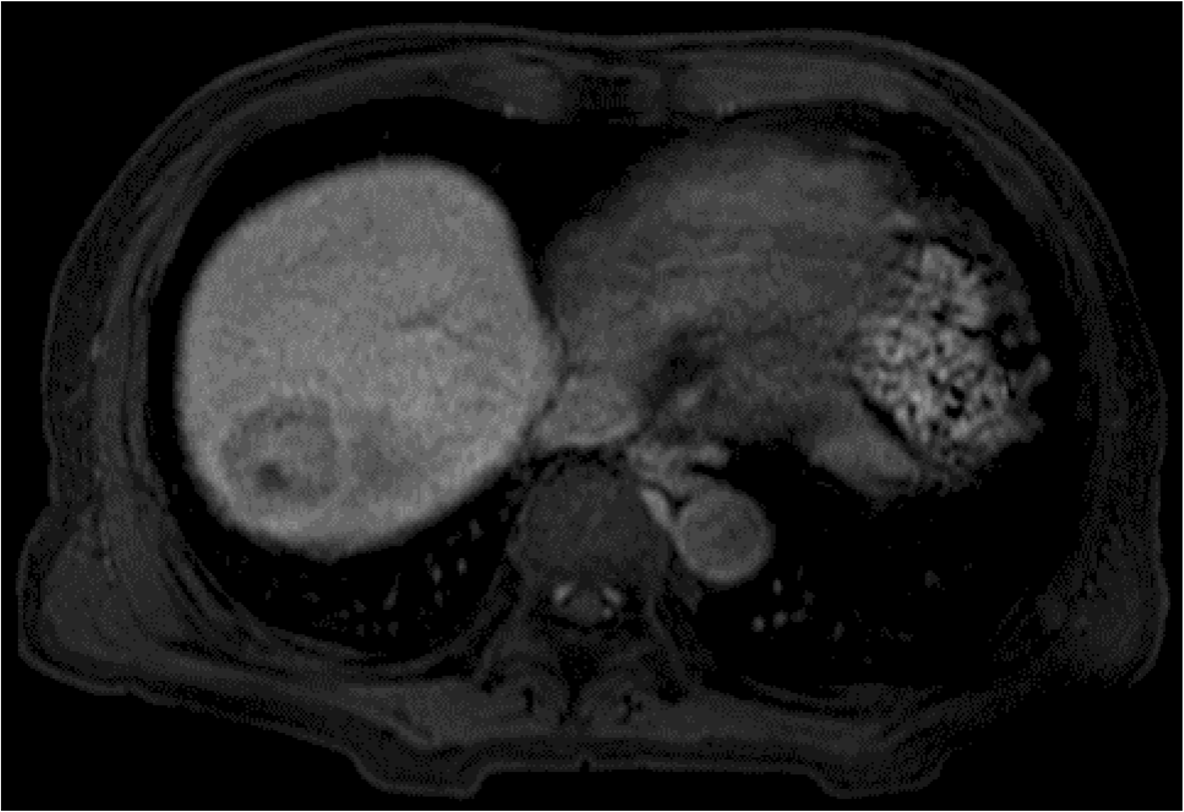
Magnetic resonance imaging (MRI) at 15 min since contrast injection. MRI enhanced with gadoxetate sodium showing a faint uptake of the contrast medium at the central area of the hepatic mass
